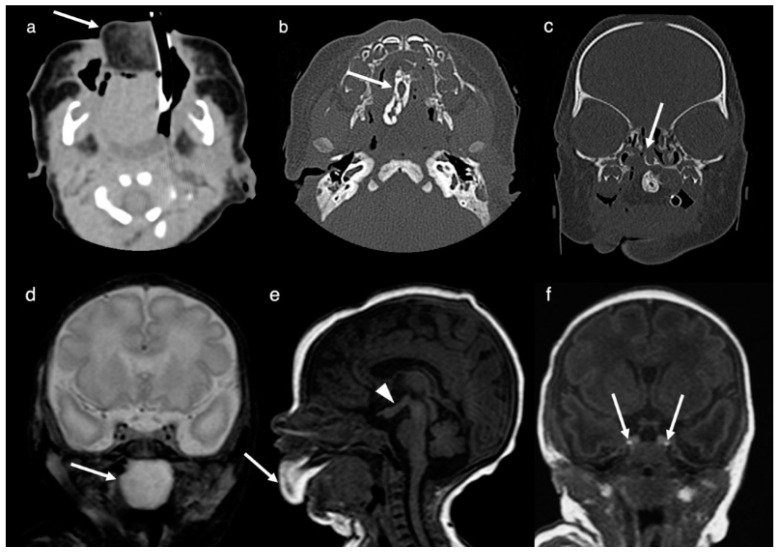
Images obtained at the second day of life. Axial CT-image (a), axial CT-image (bone algorithm, (b)), coronal CT-image (bone algorithm, (c)), coronal T2-weighted MR-image (d) and sagittal T1-weighted MR-image (e) show a heterogeneous mass centered in the oral cavity and protruding anteriorly through the mouth. The mature teratoma is composed out of fatty tissue anteriorly (arrows in (a,e)), a mineralized/bony component in the midline (arrow in (b)) and a cystic component posteriorly within the naso-/oropharynx (arrow in (d)). A cleft palate with corresponding defect in the hard palate can be seen in (c) (arrow). The tuberomammillary fusion is shown in (e) (arrowhead). Coronal T1-weighted MR-image (f) documents a complete duplication of the pituitary gland including the pituitary stalk. The bright spots of the duplicated posterior pituitary are clearly depicted (arrows in (f)).
